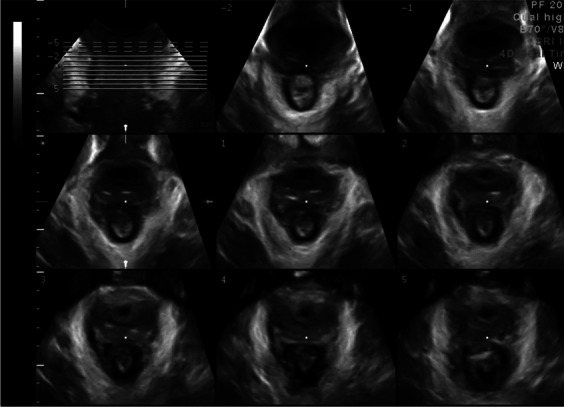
Tomographic ultrasound imaging assessment of the levator ani muscles Intact LAM [8, 14]. (Republished with permission of John Wiley & Sons—Books, permission conveyed through Copyright Clearance Center, Inc)
